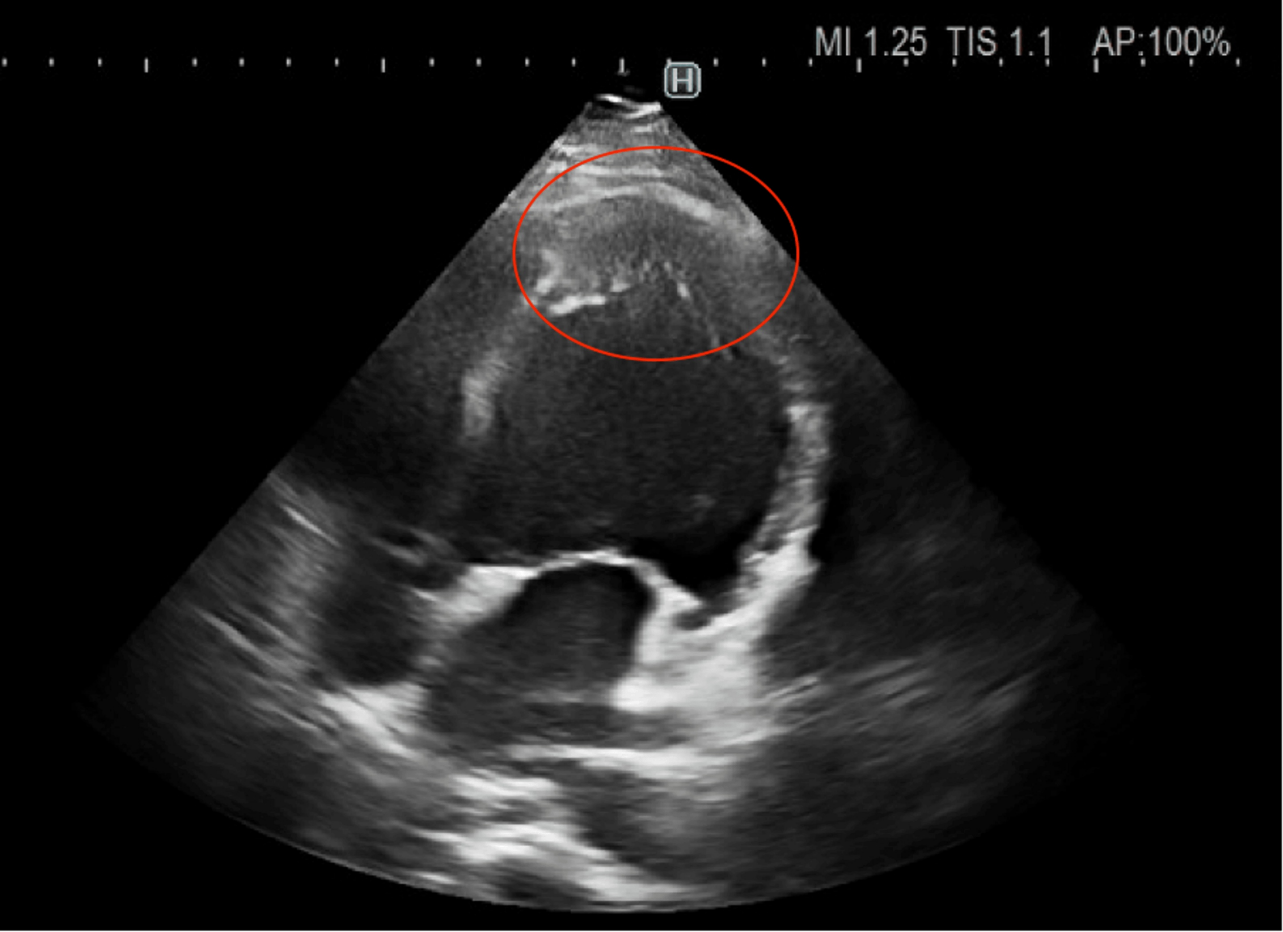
Apical four-chamber view showing a thickened apex of the left ventricle
